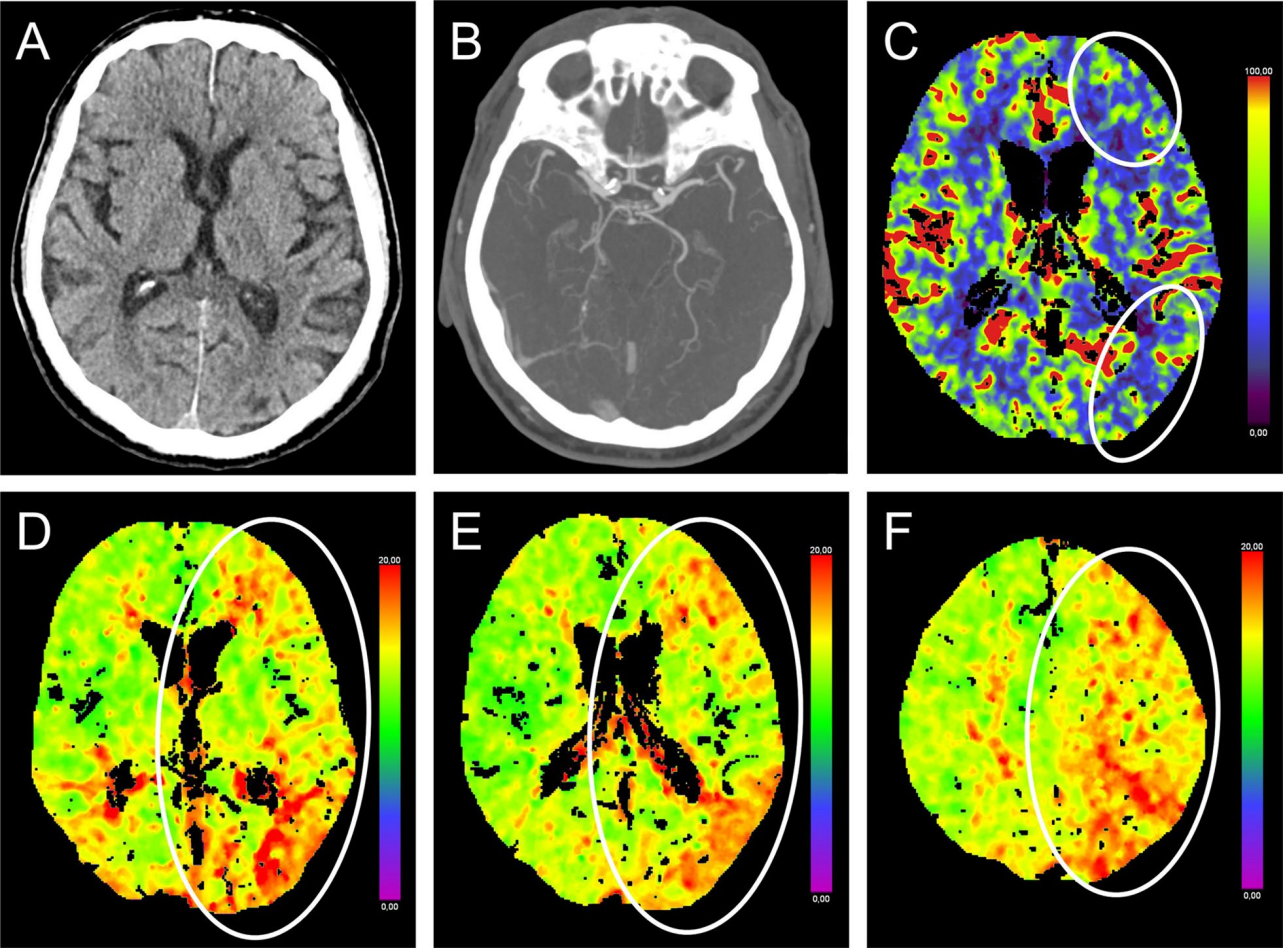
NcCT, CTA, and CTP of patient 2. Exemplary cuts of first ncCT (A), CTA (B), and CTP (C–F) 70 min after onset of symptoms. CTA without relevant stenosis but with elongation of extra- or intracranial vessels (B). Follow-up ncCT 24 h after onset (not shown) did not show infarct demarcation compared to first ncCT (A). Hypoperfusion in left frontal and left parieto-temporo-occipital areas on TTP with Tmax of > 6 s (D–F) and smaller reduced perfusion on CBF (C) marked with circle. CBF, cerebral blood flow; CT, computed tomography; CTA, CT Angiography; CTP, CT Perfusion; ncCT, non-contrast CT; Tmax, time-to-maximum; and TTP, time-to-peak
